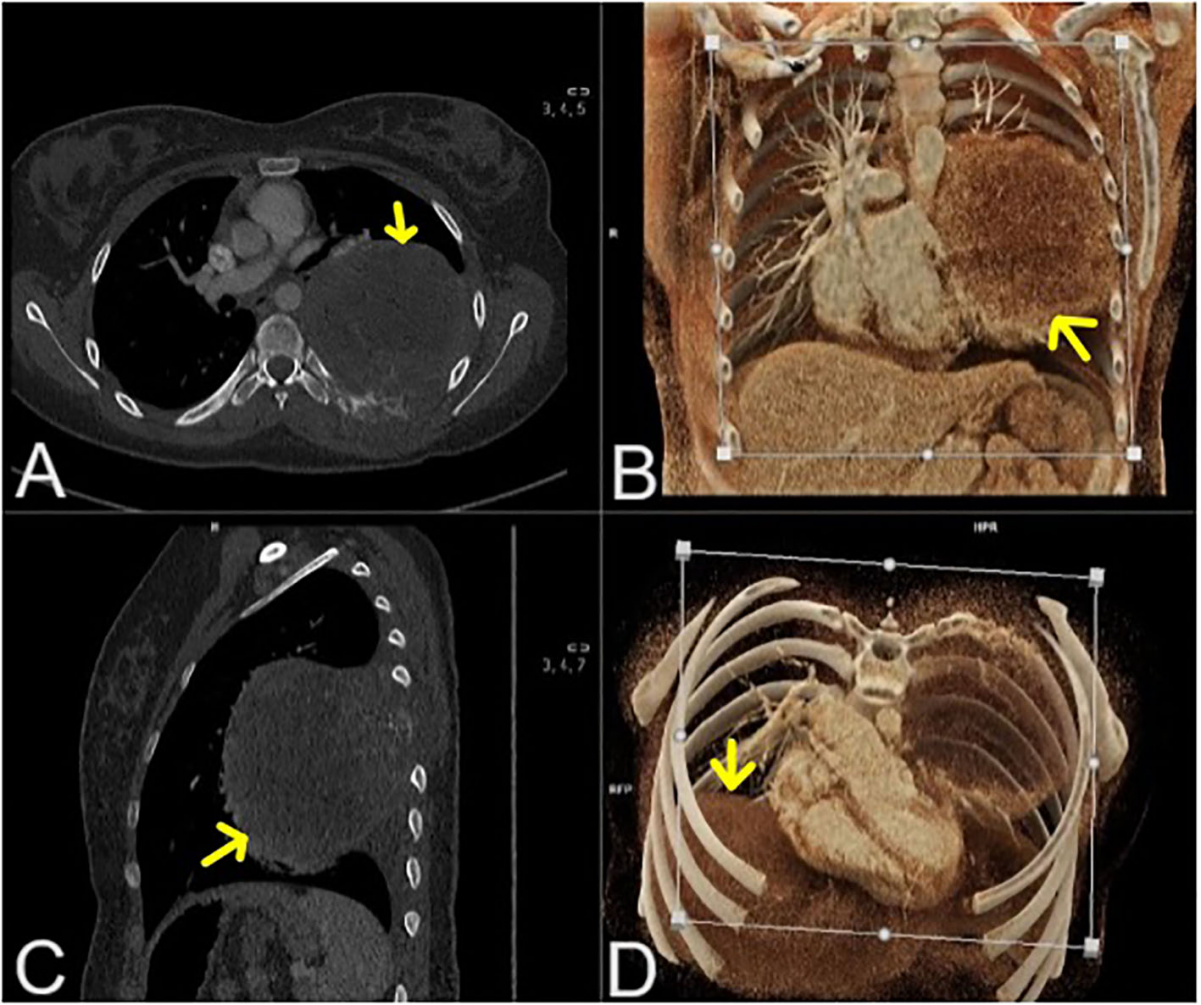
Chest computed tomography (mediastinal window after contrast agent administration- transverse [multiplanar reconstruction (A), volume rendering technique reconstruction (D)], coronal [volume rendering technique reconstruction (B)] and sagittal [multiplanar reconstruction (C) section]. Primary tumor mass marked with yellow arrows.
